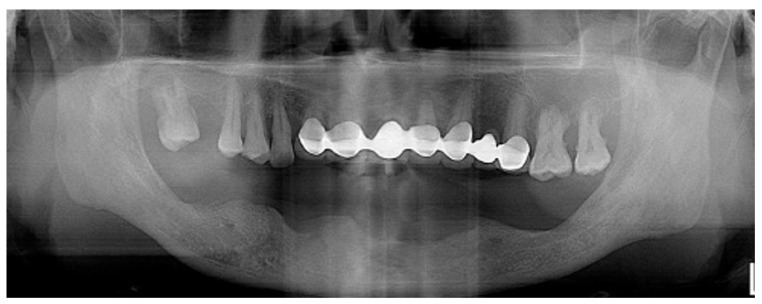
The preoperative panoramic radiograph reveals generalized moderate to severe alveolar bone resorption in the mandible, with particularly pronounced bone defects observed in the left posterior region.
